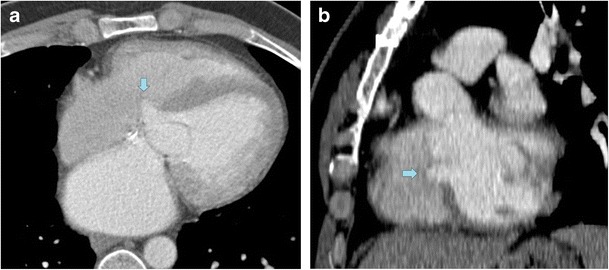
26-year-old man with a history of repaired ASD and atrial arrhythmias. Axial CT image (a) and oblique sagittal MPR (b) show a small IVMS outpouching into the RV (*arrows*)
